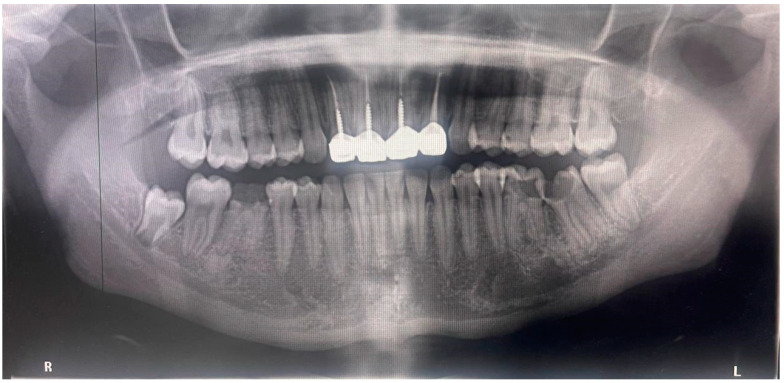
Orthopantomogram showing badly decayed lower left first and second molar.
